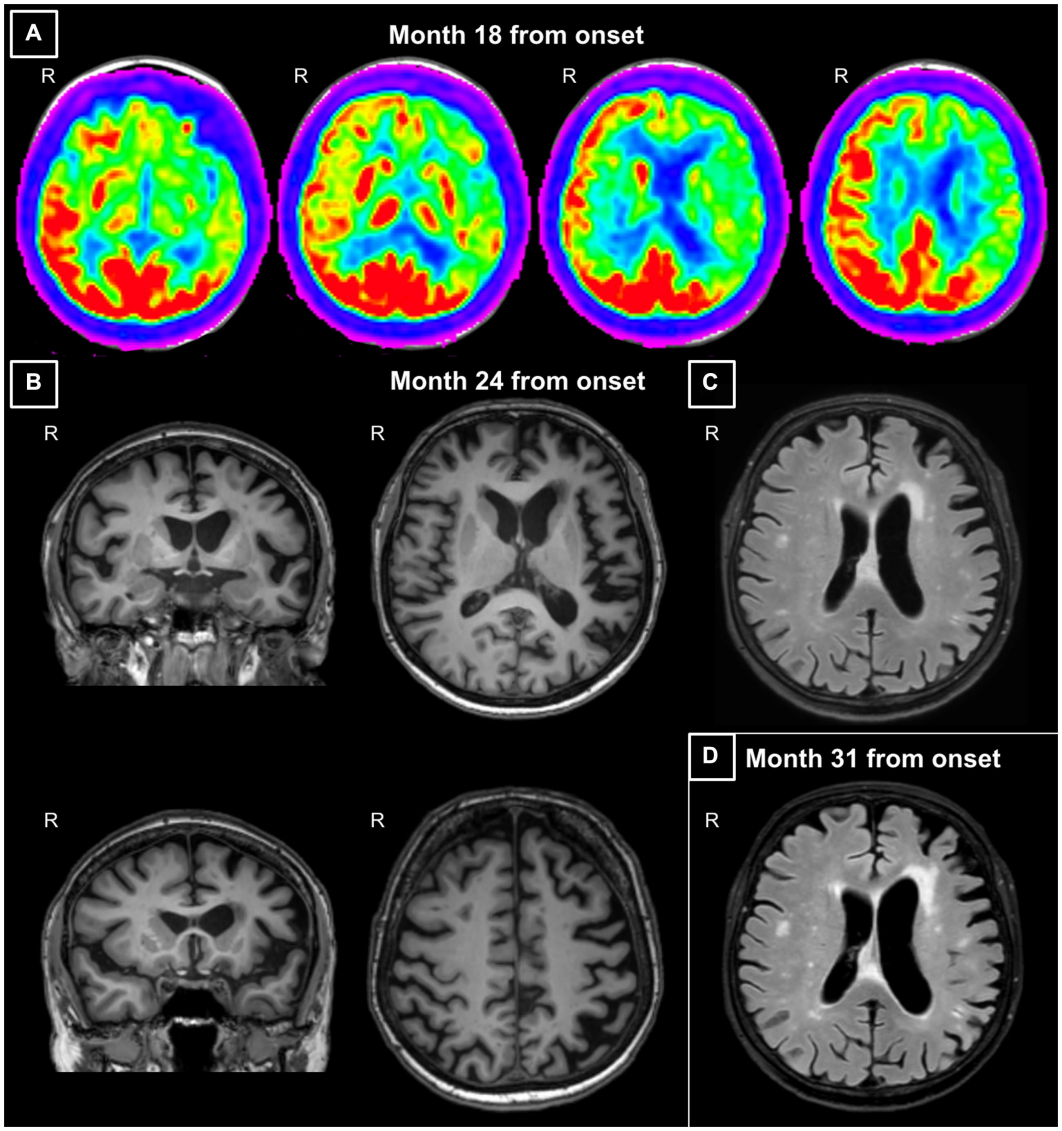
(A) Axial views of the fluorodeoxyglucose (FDG)-positron emission tomography (PET) acquired at month 18 from symptom onset. Images show hypometabolism in the left fronto-insular, fronto-temporal and temporo-lateral regions, and in the basal-ganglia with a left predominance. (B) Coronal and axial views of the 3DT1-weighted MR images acquired at month 24 from symptom onset. Images show left sylvian-perisylvian, fronto-opercular, parietal and striatal atrophy. (C) Axial view of the FLAIR image acquired at month 24 from symptom onset. The image shows prevalent left frontal periventricular white matter hyperintensities. (D) Axial view of the FLAIR image acquired at month 31 from symptom onset. The image shows a progressive ventricular enlargement mainly at the left side and increased burden of left frontal white matter hyperintensities. Images are displayed according to standard radiological convention. R, right.
